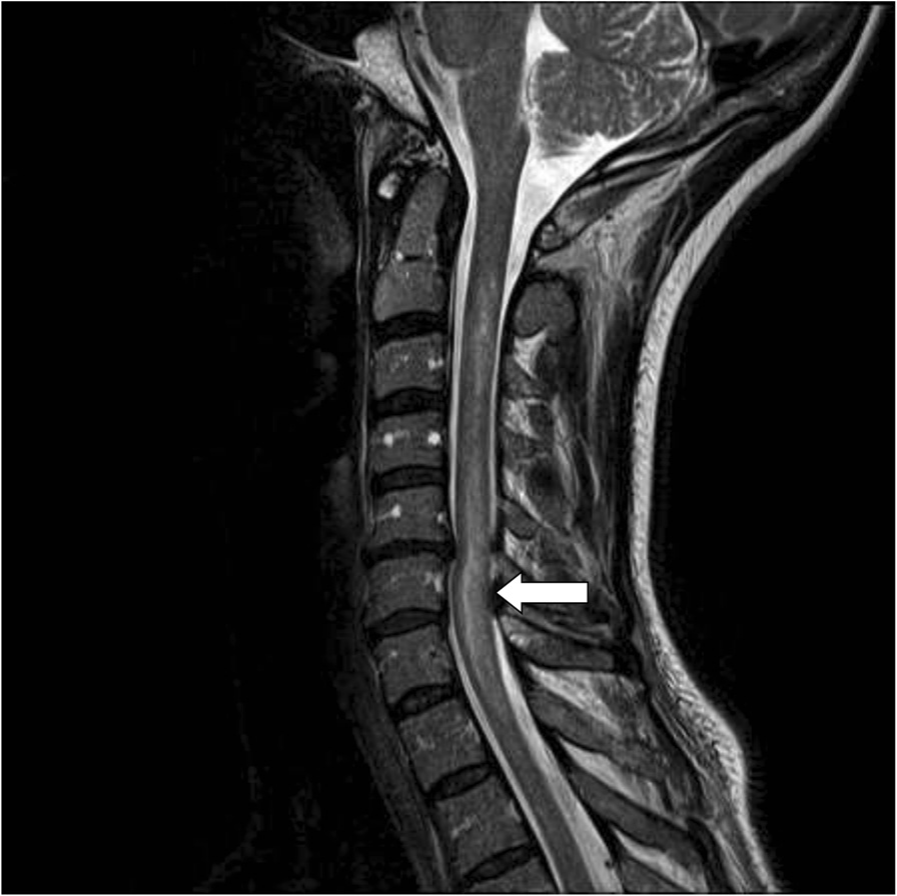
MRI T2 weighted sequence of the cervical MRI showing abnormal signal changes extending from C2-T1 with worsening enhancement at lower cervical cord levels.
